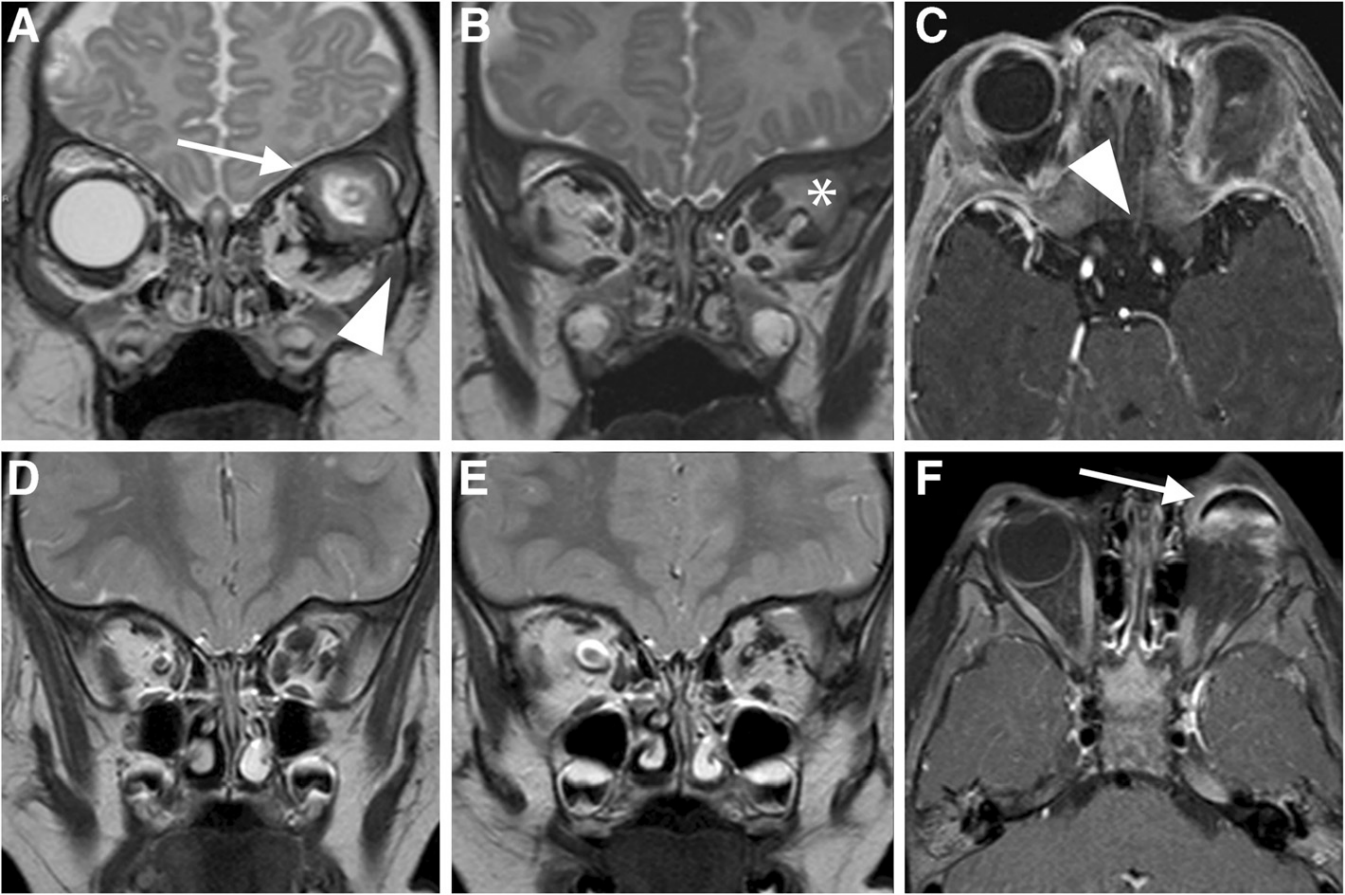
Pre-operative (A-C) and post-operative (D-F )orbit MRI study. Coronal T2w images (A-B) show a complex mass in the upper-outer and anterior portions of the left orbit with a predominantly extraconal engagement. The mass consists of a well-defined anterior cystic component (white arrow) with signal intensity similar to vitreous/cerebrospinal fluid (CSF) and a posterior component more hyperintense than the muscle signal. No normal orbital contents such as extra-ocular muscles or globe can be identified in the anterior portions of the orbit. Axial post-gadolinium fat saturated T1w image (C) shows heterogeneous enhancement of the posterior soft tissue mass. No normal orbital contents such as extraocular muscles or globe can be identified in the anterior portions of the orbit. Notice the bone defect of the lateral wall of the orbit (A, arrowhead) and the left optic nerve atrophy (C, arrowhead). Post-operative Coronal T2w MRI images (D-E) disclose a partial reduction of the complex mass in the left orbit; following gadolinium administration, the residual lesion shows a dyshomogeneous enhancement (axial post-gadolinium fat saturated T1w image, F); notice the presence of a left ocular prosthesis (F, white arrow).
